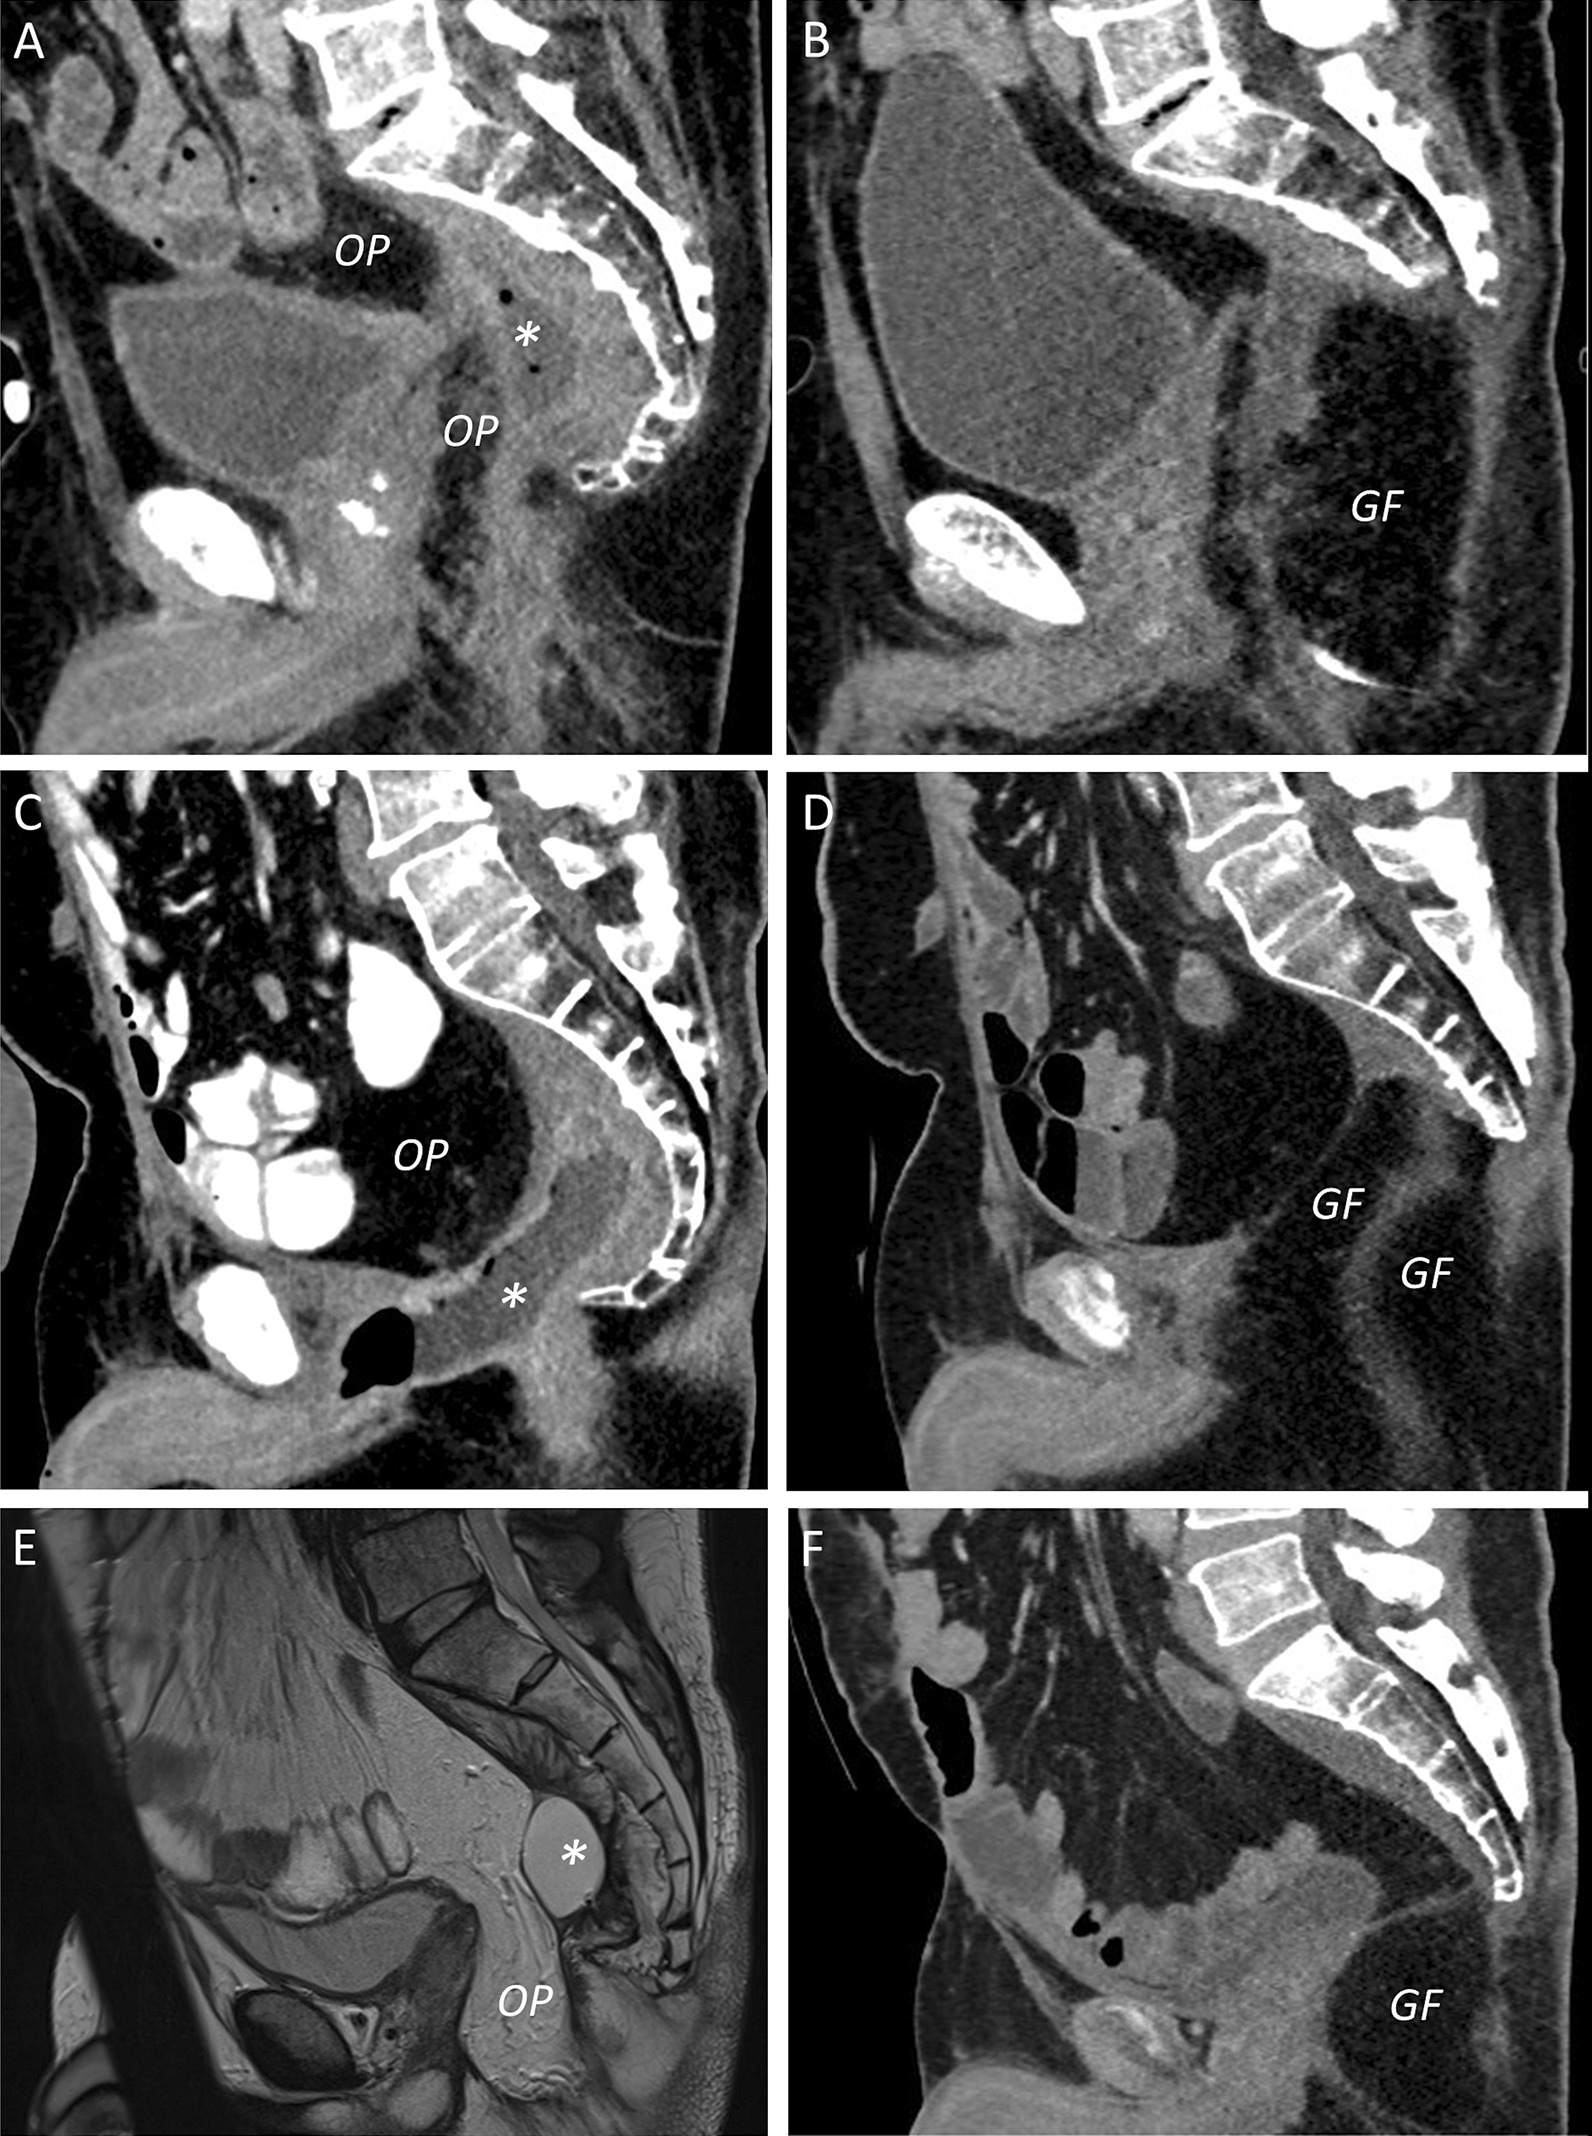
Pre- and postoperative sagittal pelvic imaging using CT (a–d, f) or MRI (e), showing the recurrent and persisting abscess (*) despite omentoplasty (OP) and drainage procedures, and subsequent postoperative result after distal sacrectomy with gluteal V–Y fasciocutaneous advancement flap (GF) in the first (a, b), second (c, d), and third patient (e, f). Some residual fluid below the flap was observed in the first patient (b), which was successfully drained
